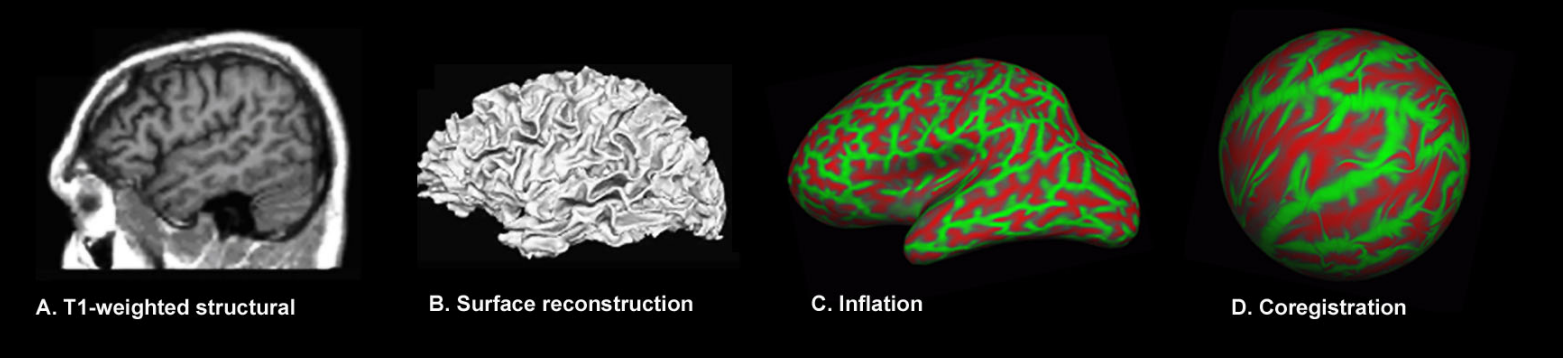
SBM image processing steps: Surface reconstruction and alignment to standard template. A. High-resolution T1-weighted images were processed using FreeSurfer 3.0 to map the cortical surface. The image is a lateral view of the TBI patient's left hemisphere. B. Computerized reconstruction of the gray/white matter boundary. A smoothed and expanded view of the white matter surface is shown. The image has been intensity normalized, skull-stripped and the cerebellum has been removed. C. Inflation of the cortical surface to map gyral and sulcal anatomy. Gyral regions are shown in green and sulcal regions in red. D. Coregistration of the subject's cortical surface to a common spherical template. This step allows the assessment of cortical tissue properties with respect to a normative database using a common coordinate system.
